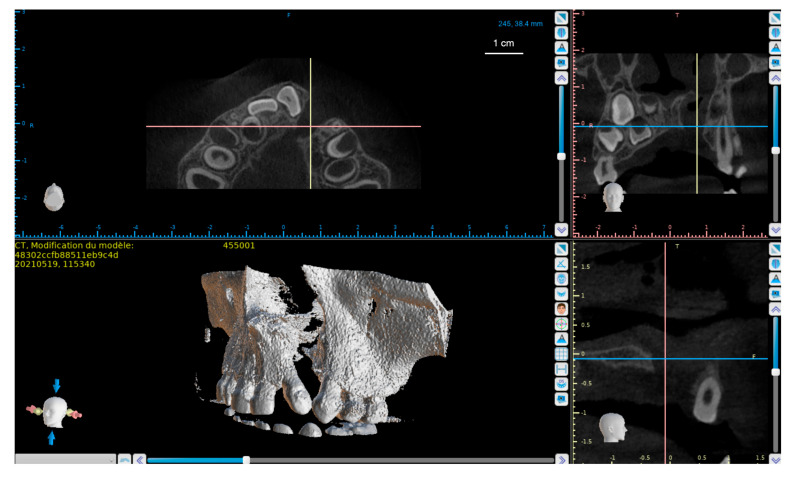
Top left: coronal section of a CBCT of a patient with a cleft alveolar-palate. The two fractures are perfectly individualized. Bottom left: volume modeling from DICOM files before export.
